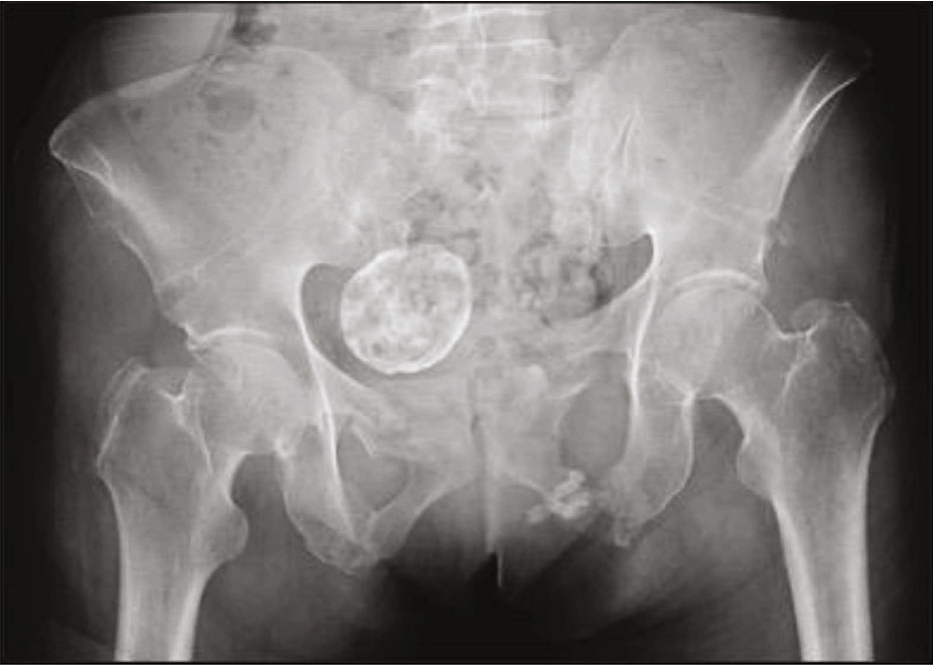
Anteroposterior pelvis radiograph after removal of the external fixator with healing of pubic rami fractures and presence of the antibiotic beads.
